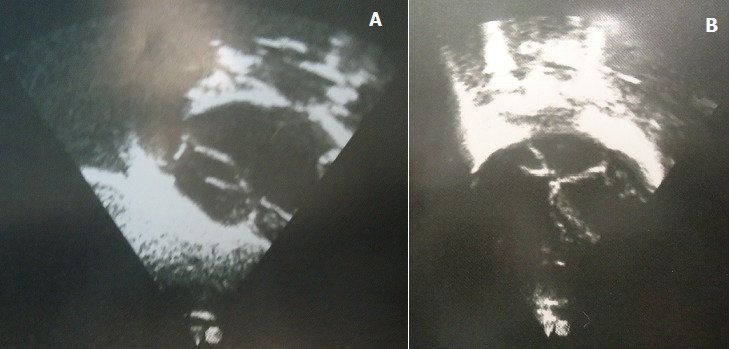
(A) subcostal view and (B) apical four chamber view of transthoracic echocardiography of the patient, showing a thick band of echogenic tissue dividing the right atrium into 2 unequal halves
